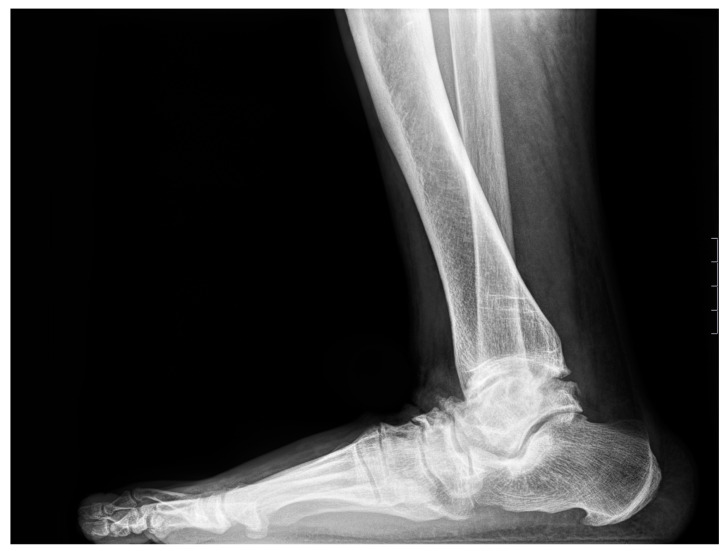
Lateral radiograph of the foot: 50-year-old female patient presenting with enthesopathies of the talus and navicular bones and severe JSN of the TN and NC joints.
